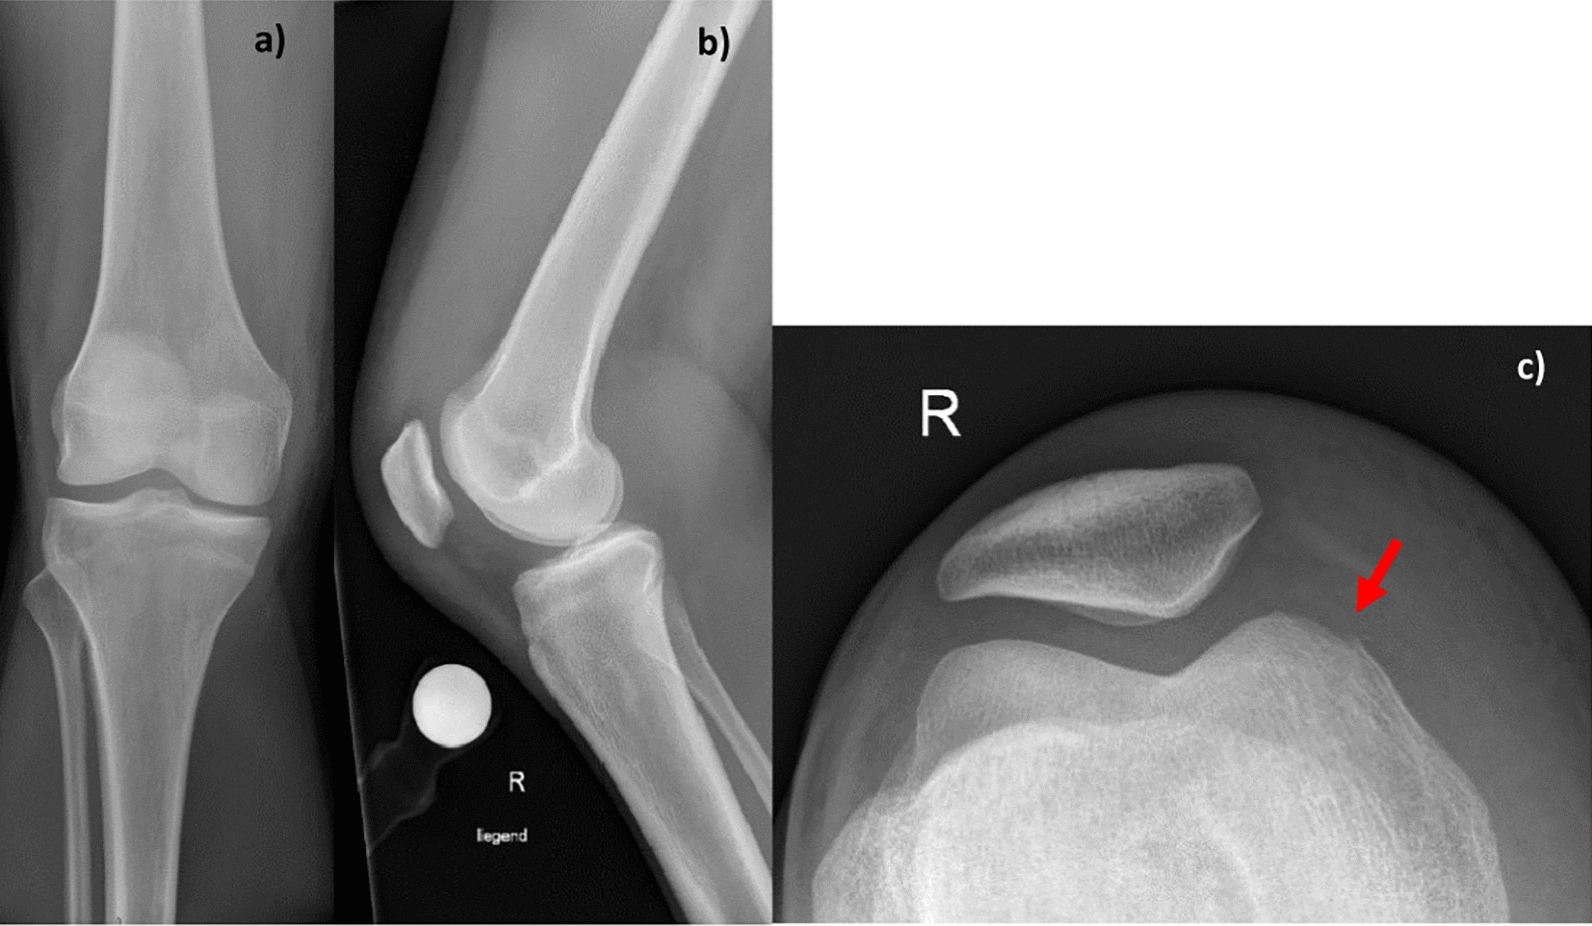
The radiographs of right injured knee taken at the initial appearance at our emergency department to see joint effusion and to rule out fracture. a Shows the anteroposterior view; b the lateral view; and c the tangential patella view. On image c, you can see the irregularity of the corticalis at the medial femur condyle (red arrow)
